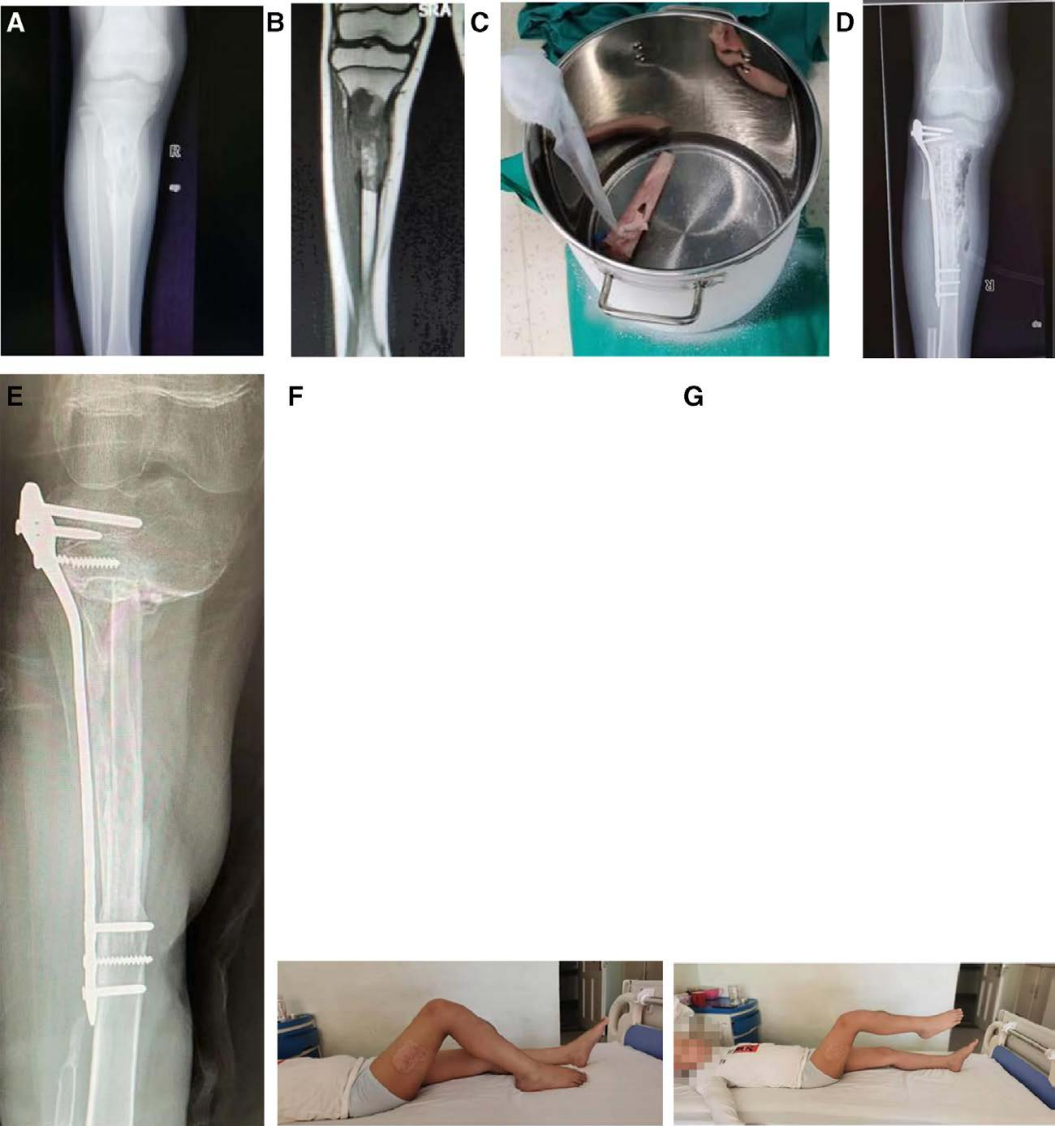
(A) The preoperative X-ray. (B) The preoperative MRI. (C) Autologous bone liquid nitrogen inactivation. (D) Postoperative X-ray. (E) X-ray showed 2 years after the operation. (F and G) The patient’s lower limb function was satisfactory. MRI = magnetic resonance imaging.
